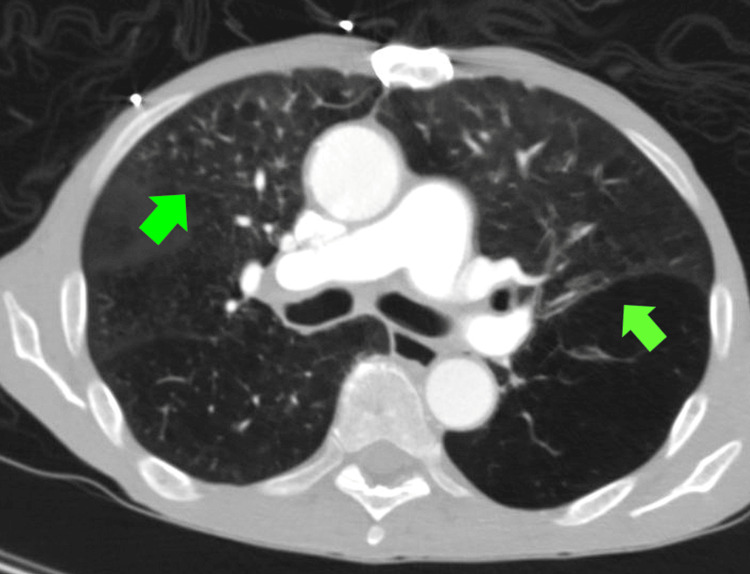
Transverse chest CT angiography (CTA) showing chronic severe emphysema and bronchitis (green arrows).
